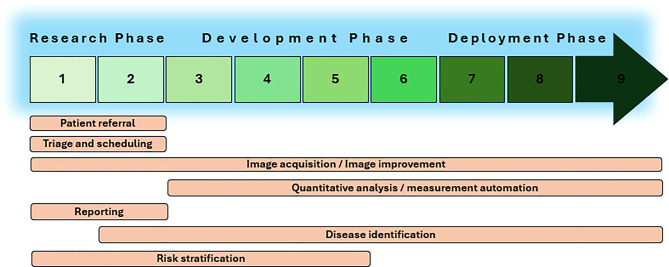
The readiness level of AI models within echocardiograph
